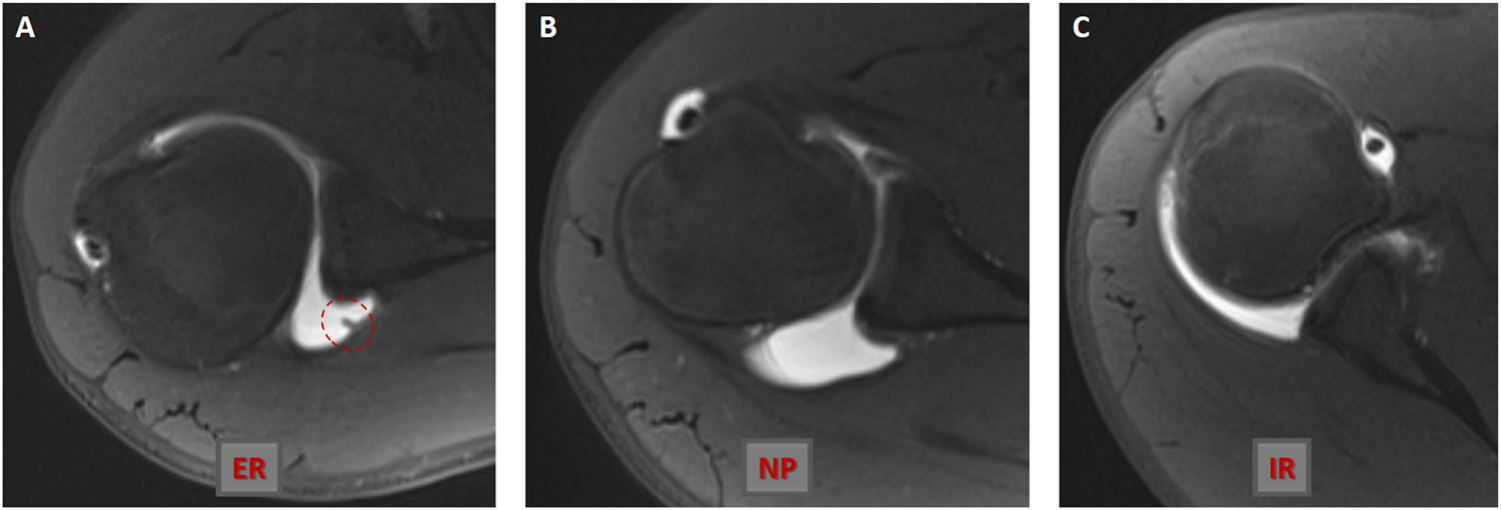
Axial fat-suppressed TSE T1-weighted MR arthrogram (A) obtained in the external rotation of the ipsilateral arm shows a synovial plication (circle) in the posterior shoulder joint capsule. Axial fat-suppressed TSE T1-weighted MR arthrography sections (B, C) passing through the same level as in Fig. 1A show normal anatomy of the posterior shoulder joint capsule in the neutral position and internal rotation of the ipsilateral arm. ER, external rotation; IR, internal rotation; NP, neutral position
